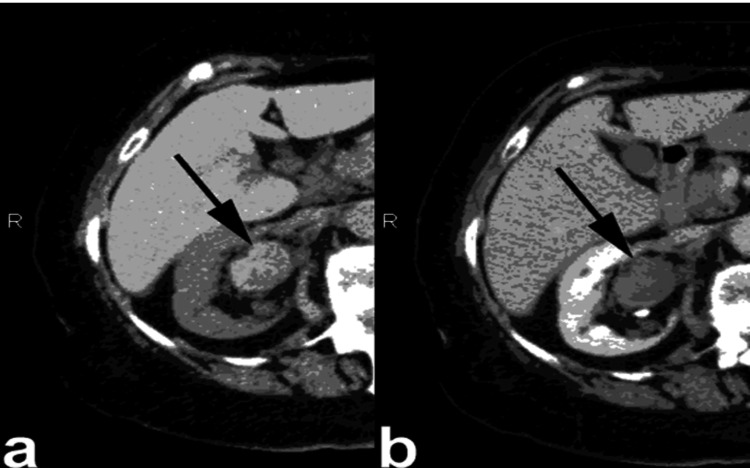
Imaging studies comprising non-enhanced CT (NECT) (a) and contrast-enhanced CT (CECT) during the urographic phase (b). These modalities were utilized to evaluate a rare complication associated with blood thinners: a right kidney sinus hematoma. The NECT scan (a) provided an initial assessment, revealing the presence of a hematoma within the right kidney sinus. Subsequent CECT during the urographic phase (b) further delineated the extent and characteristics of the hematoma, aiding in its precise characterization and assessment of potential complications. This figure is a republished image, permission was obtained from the original publisher, https://epos.myesr.org/poster/esr/ecr2018/C-2802
